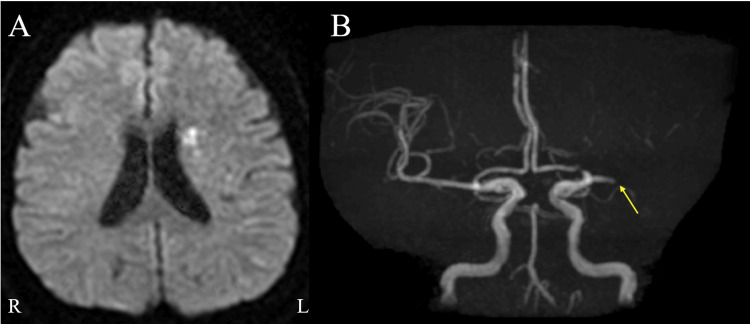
The results of brain MR imaging on admission (A) Diffusion-weighted brain MR imaging showing hyperintensity in the left corona radiata region. (B) MR angiography showing occlusion of the left middle cerebral artery (arrowhead).
